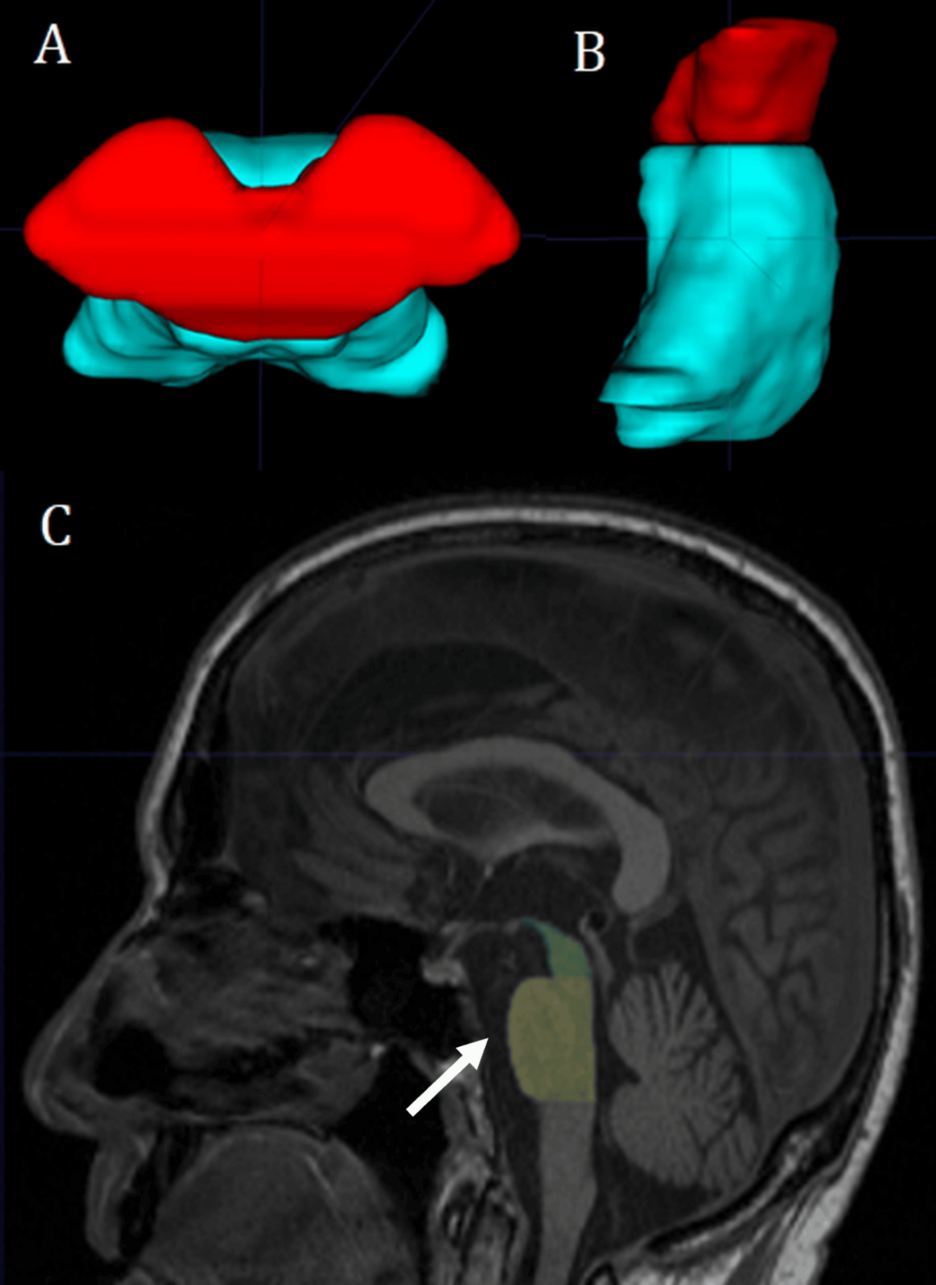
Volumetric and Morphometric MRI Analysis Panel A shows the volumetric reconstruction, the midbrain is seen in red and the pons in turquoise (superior view). Panel B presents the lateral view of the reconstruction. Panel C shows a mid-sagittal T1 MRI image, the pons is labelled as yellow and the midbrain as green; note the characteristic “hummingbird sign”, named due to the thinning of the anterior aspect of the midbrain resembling the beak of a hummingbird (white arrow).
